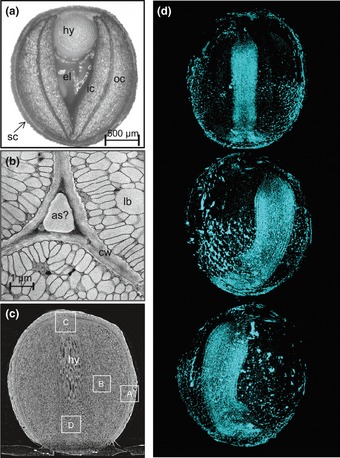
Structural organization of a developing oilseed rape (*Brassica napus*) seed using conventional and non-invasive analysis. (a) Horizontal mid section of a developing seed sampled *c*. 30 d after flowering. (b) A TEM acquired image shows the presence of lipid bodies inside the cotyledonary cells and extracellular voids at the cell corners. (c) A high resolution CT vertical radial section of a whole rapeseed (1.7 μm per pixel). A fuller visualization of these images is provided in Supporting Information Movie S1. The rectangles indicate regions modelled in Figs 2 and 3. (d) A rotation series of views of the void spaces in a seed obtained from high resolution CT. An animated three-dimensional model is given as Movie S2. as, airspace; cw, cell wall; el, endospermal liquid; hy, hypocotyl; lb, lipid body; ic, inner cotyledon; oc, outer cotyledon; sc, seed coat (testa).
